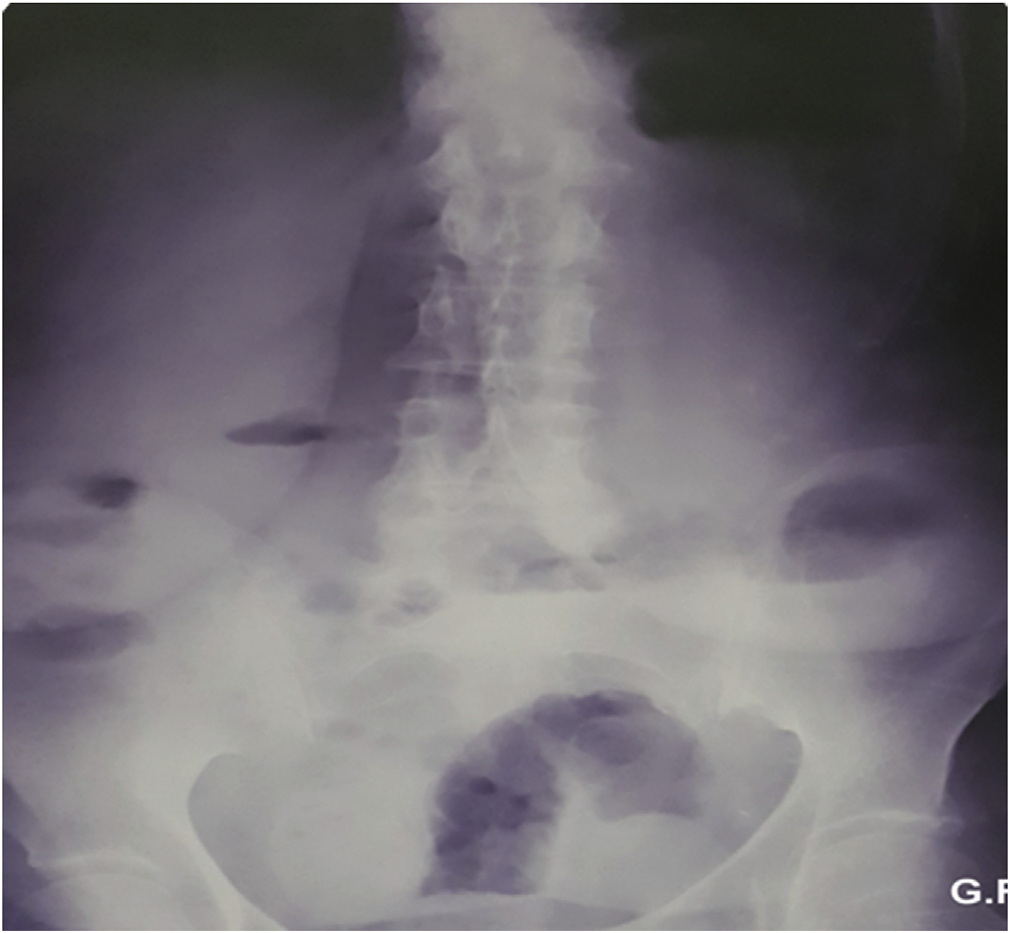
Plain Abdominal X-ray showing air-fluid levels with air in the rectum.
